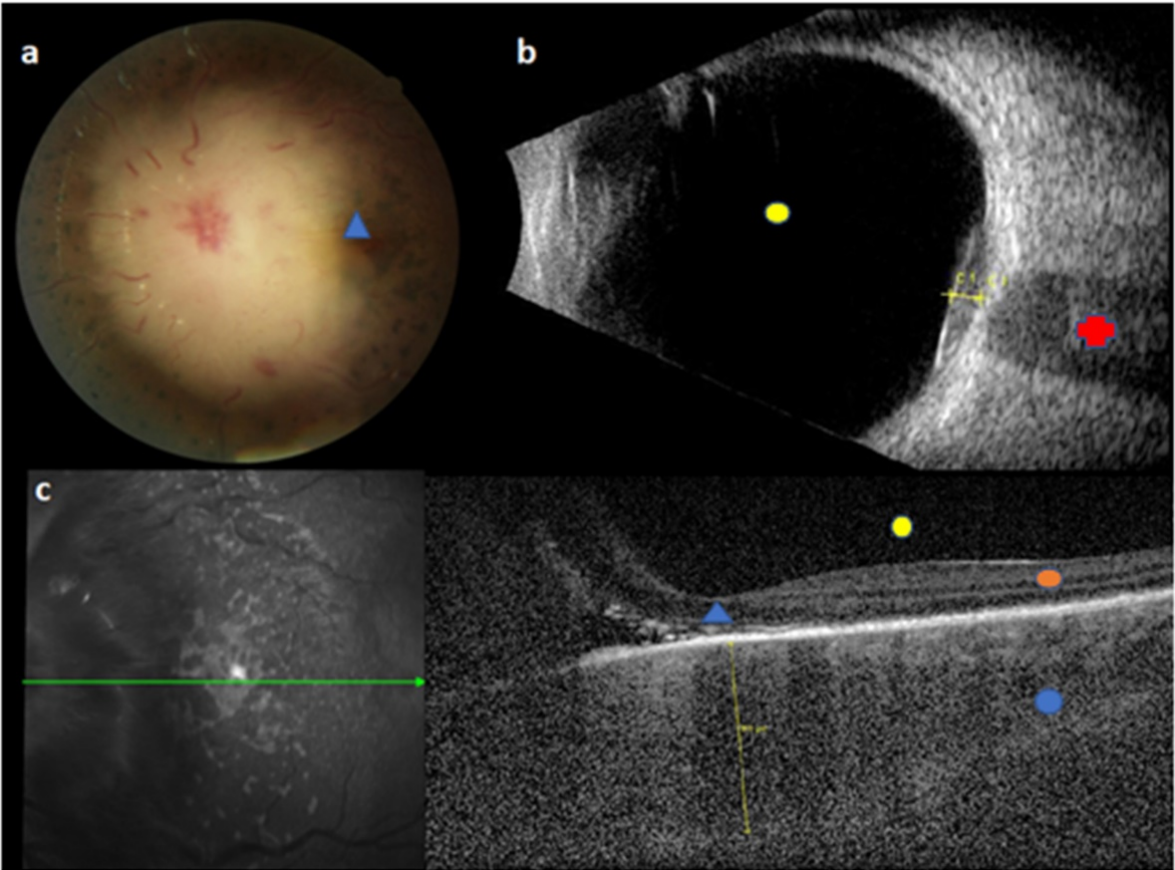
Initial images of BCP-ALL ophthalmic relapse for a child with normal brain imaging and no blast at CSF. This figure reveals a sub-macular choroidal hypertrophy and choroidal infiltration above the optic nerve. a. Fundus retinography: choroidal infiltration above optic nerve; all big papillary vessels and papilla are hidden by the choroidal infiltration, blue triangle = macula. b. B-ultrasonography: C1 = infiltration above optic nerve; red cross = optic nerve; yellow circle = vitreous cavity. c. left picture: fundus retinography; green arrow = horizontal line of OCT. Right picture: horizontal OCT: yellow line = sub-macular choroidal hypertrophy; blue circle = choroid, orange circle = retina; yellow circle = vitreous cavity, blue triangle = macula. Legend: ALL: acute lymphoblastic leukemia; CSF: cerebrospinal fluid; OCT: optical coherence tomography.
